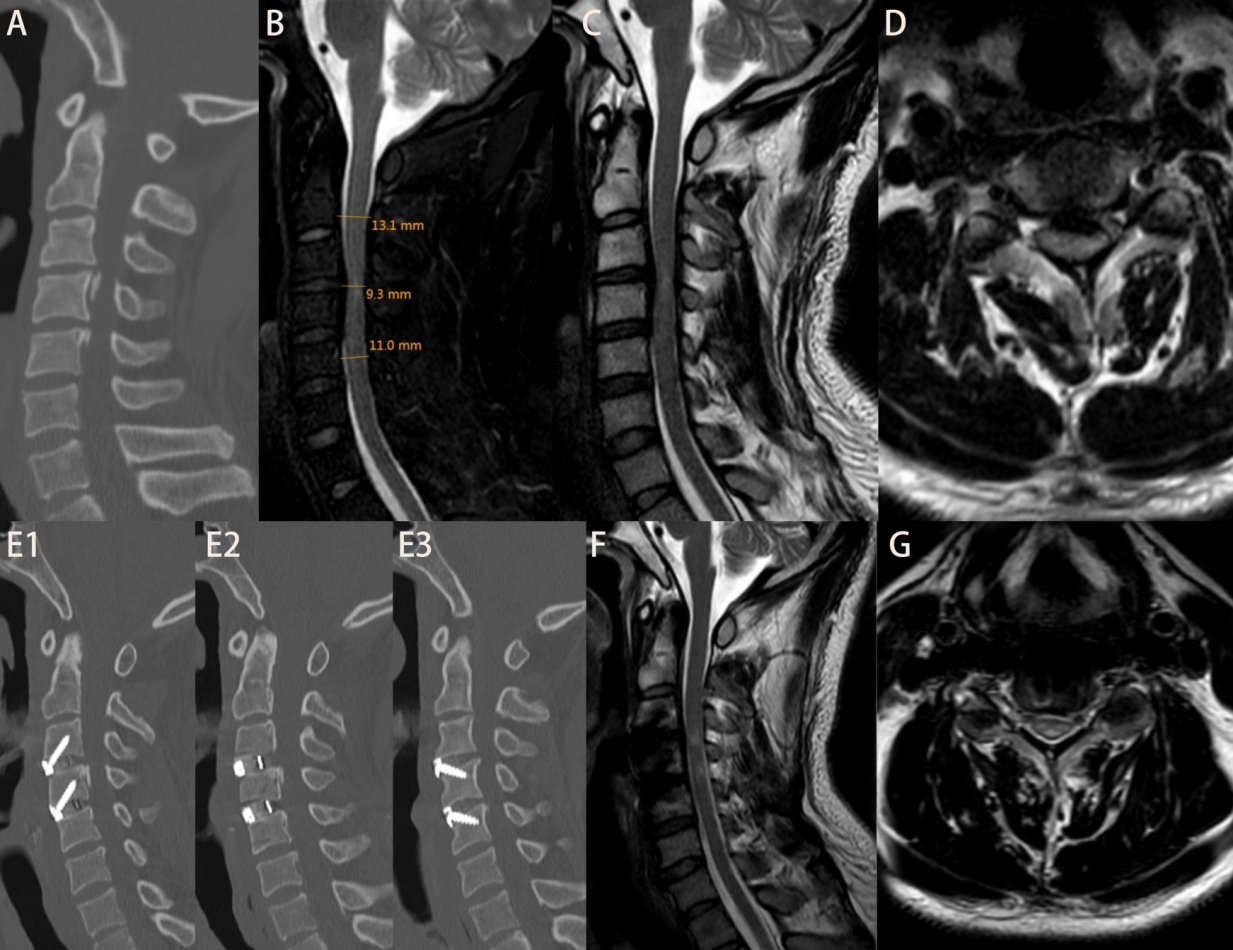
Patient Ma, admitted 6 h after a fall, with an initial JOA score of 9. (A) Admission cervical CT scan showing no evidence of fracture or dislocation. (B) Measurement of di, da, db, and MSCC values; MSCC calculated to be 22.82%. (C,D) Preoperative cervical MRI showing significant spinal cord compression. On the second day post-admission, the patient underwent ACDF at C3–4 and C4–5 levels. The patient was discharged on postoperative day 10. Figures E1–E3: cervical CT at 6 months postoperatively showing good positioning of screws and interbody fusion device. (F,G) Cervical MRI at 6 months postoperatively showing decompression of the spinal cord. At 2-year follow-up, the JOA score was 16.
